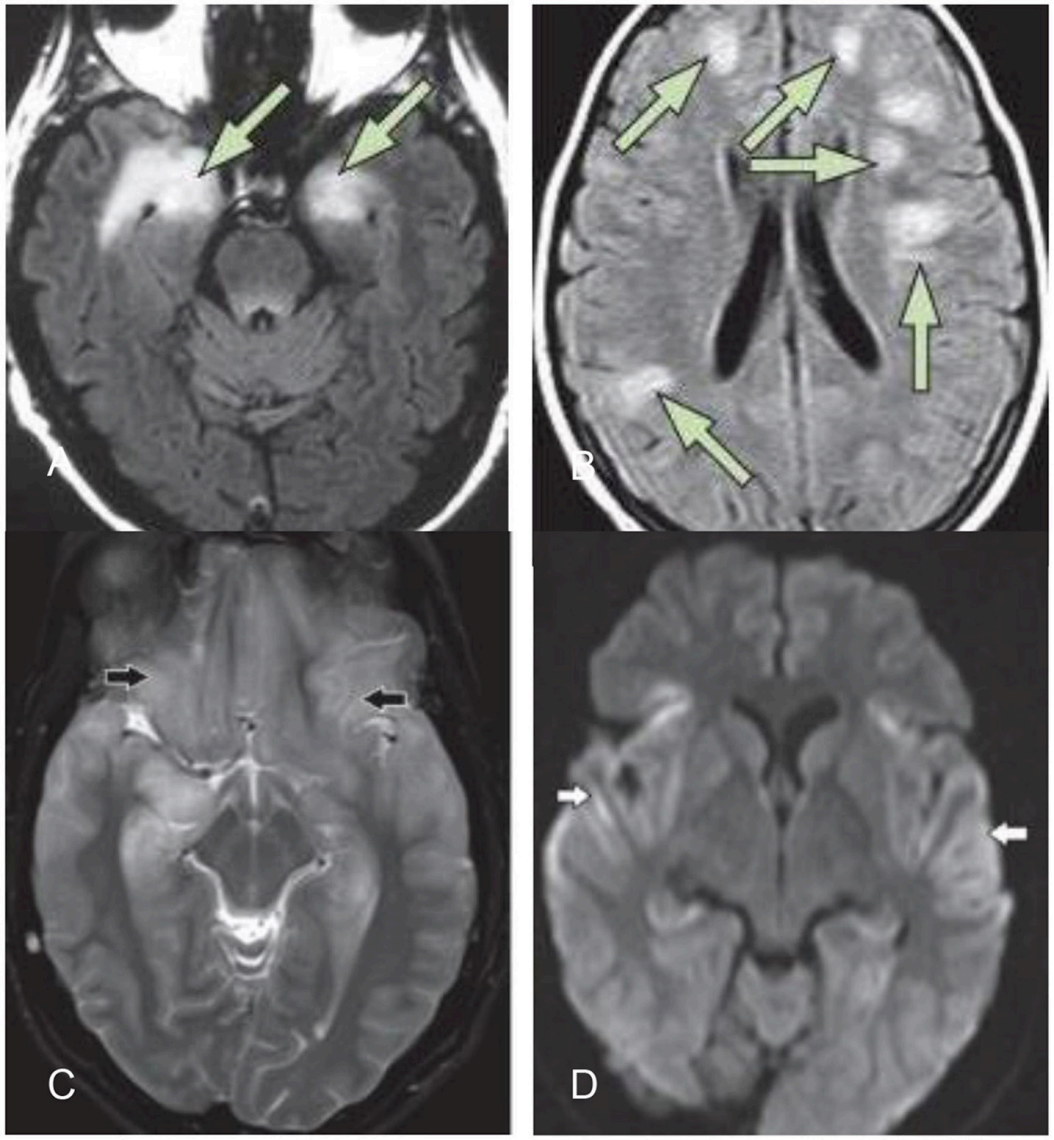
(A) Typical MRI of limbic encephalitis with bilateral hyperintensities in the medial temporal lobe on T2-weighted fluid–attenuated inversion recovery imaging (B) typical MRI of ADEM (14). (C,D) Herpes simplex virus encephalitis: bilateral symmetric cortical swelling and hyperintensity on T2 weighted image involving the anteromedial temporal lobes, insular cortex, orbital gyri (black arrows) with restricted diffusion in the involved areas (white arrows) (25).
